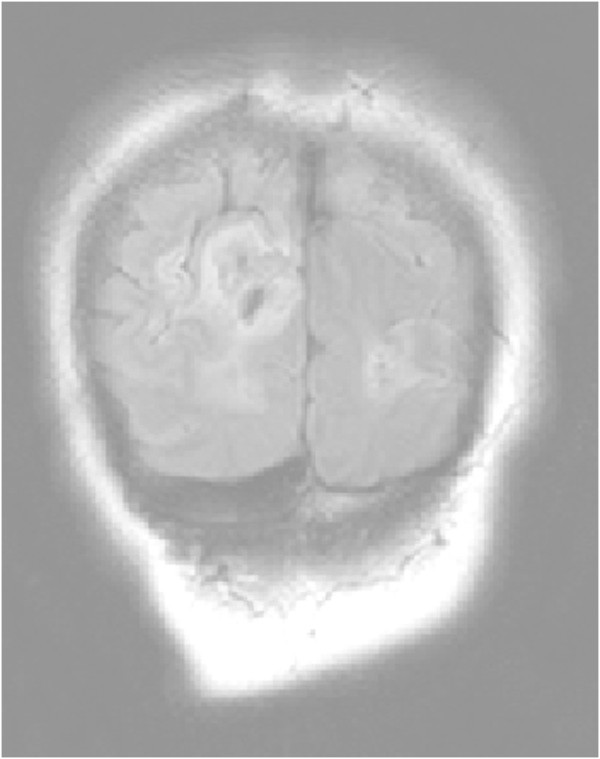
Coronal FLAIR after 5 months.
